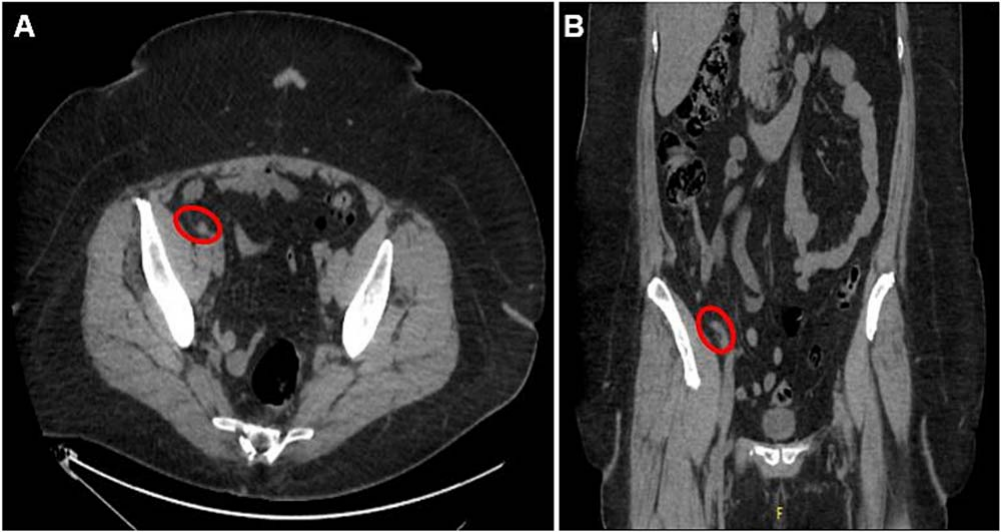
Axial (A) and coronal (B) CT abdomen and pelvis images demonstrating minimally thickened appendiceal tip measuring 7.8 mm with minimal surrounding fat stranding and no adjacent free air or fluid collection, indicating uncomplicated appendicitis.
